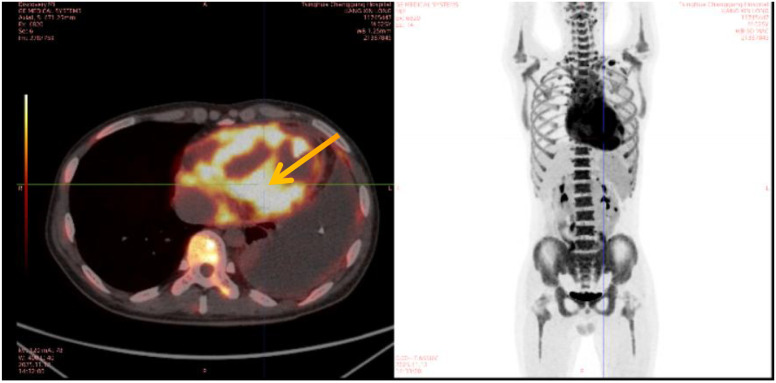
[^18^F]FDG PET–CT reveals diffuse myocardial and systemic FDG uptake. Intense myocardial FDG uptake (SUVmax 14.8) is accompanied by hypermetabolic activity in the pericardium, renal cortices, and multiple lymph node stations.
